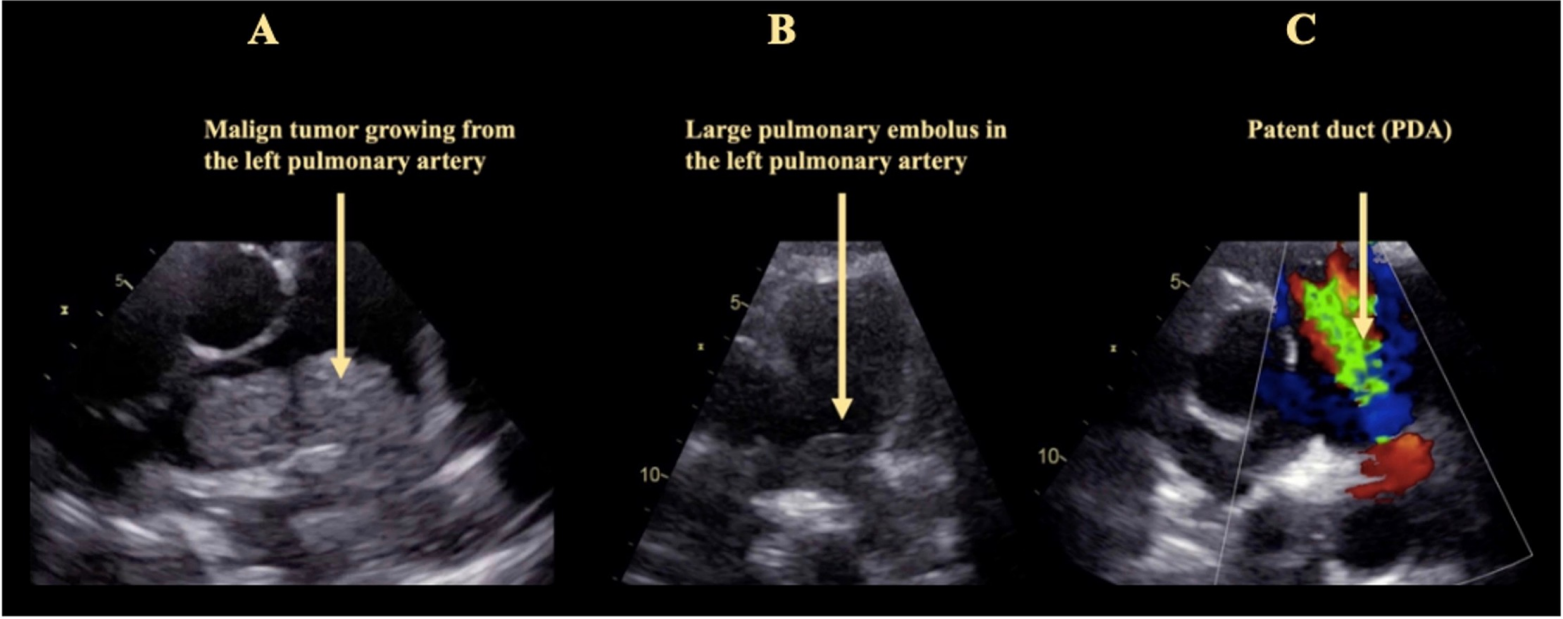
Rare findings that can be diagnosed if the pulmonary trunk is visualized routinely in transthoracic echocardiography. Panel A: A large malign tumor growing from the left pulmonary artery. Panel B: Large pulmonary embolus in the left pulmonary artery. Panel C: Patent duct.
